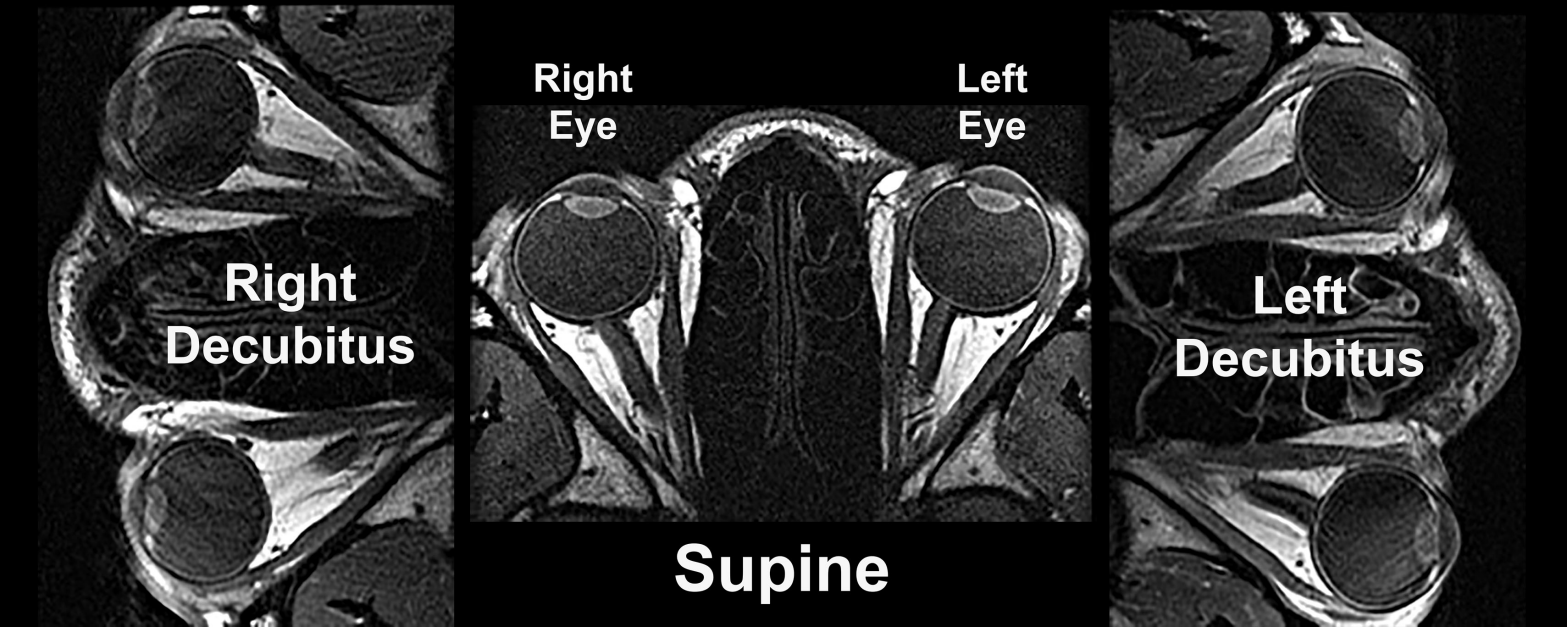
Axial T1-weighted gadolinium contrast magnetic resonance images of a normal subject in central gaze in supine and lateral decubitus positions.
